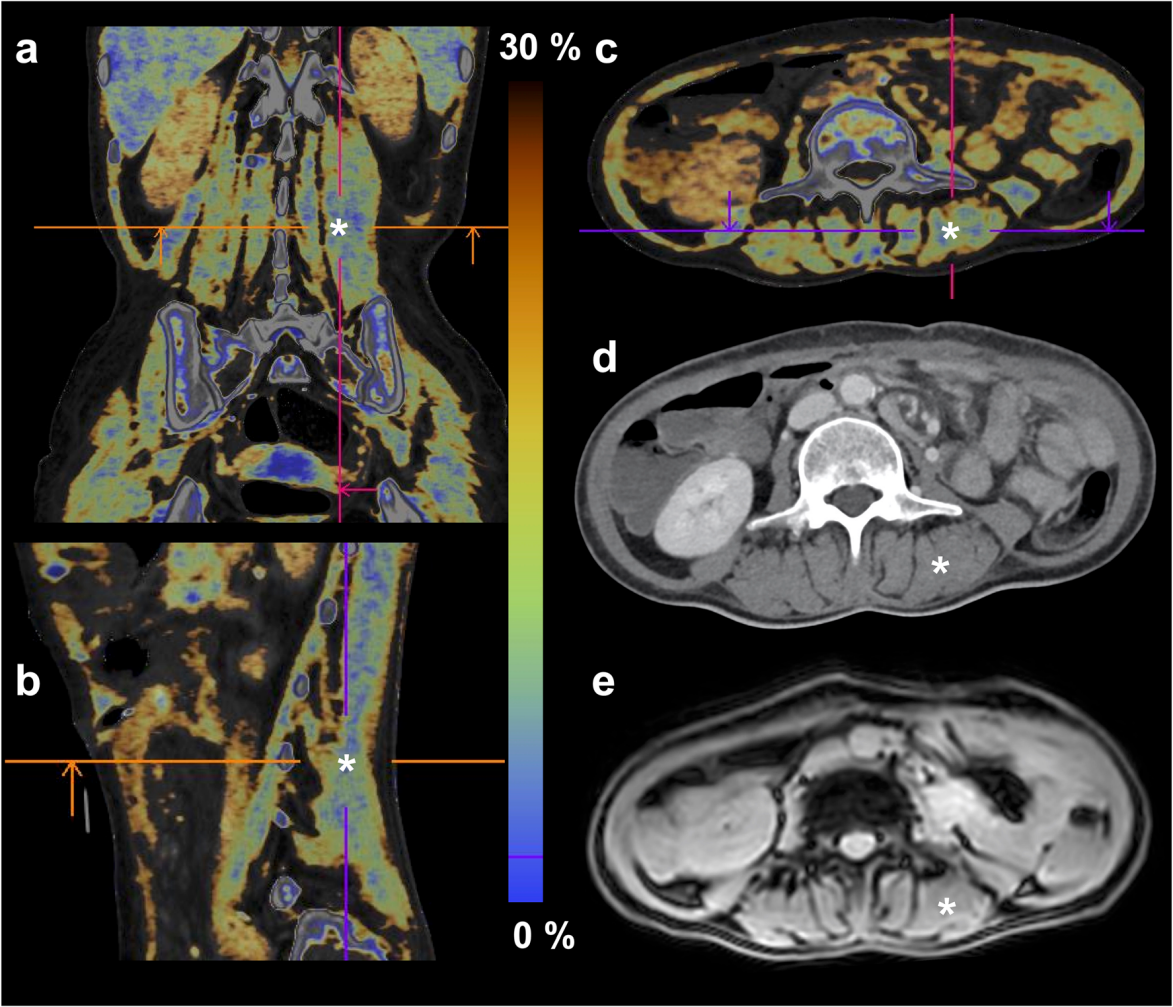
Fat map images (a–c) calculated from dual-energy computed tomography (d), and magnetic resonance magnitude image (e) of a 58-year-old female patient with non-Hodgkin lymphoma. The analyzed region of the right posterior paraspinal muscle is marked (white asterisk, cross-hairs on fat maps)
